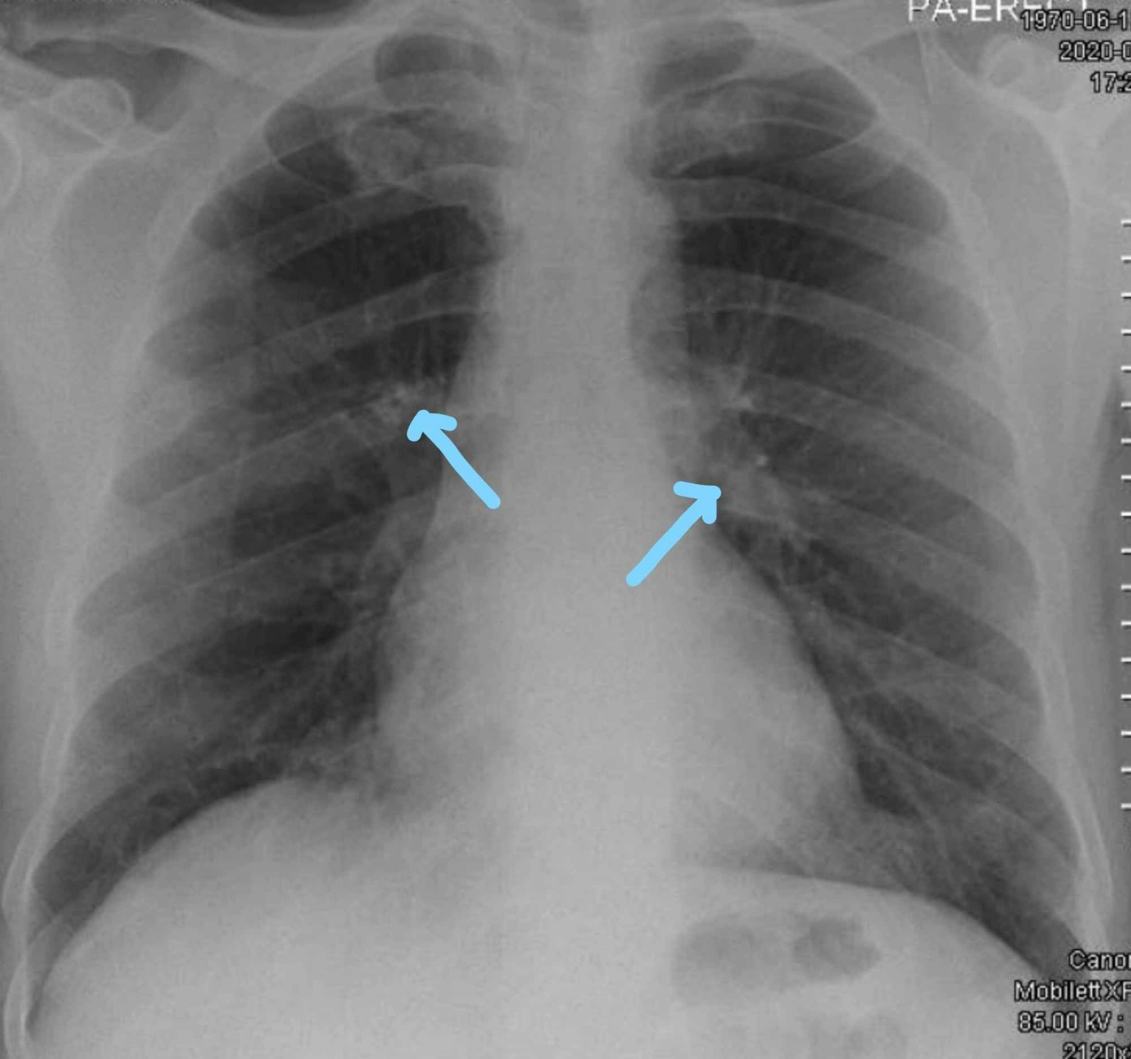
Chest X-ray on admission showing prominent bronchovascular markings and no infiltration
